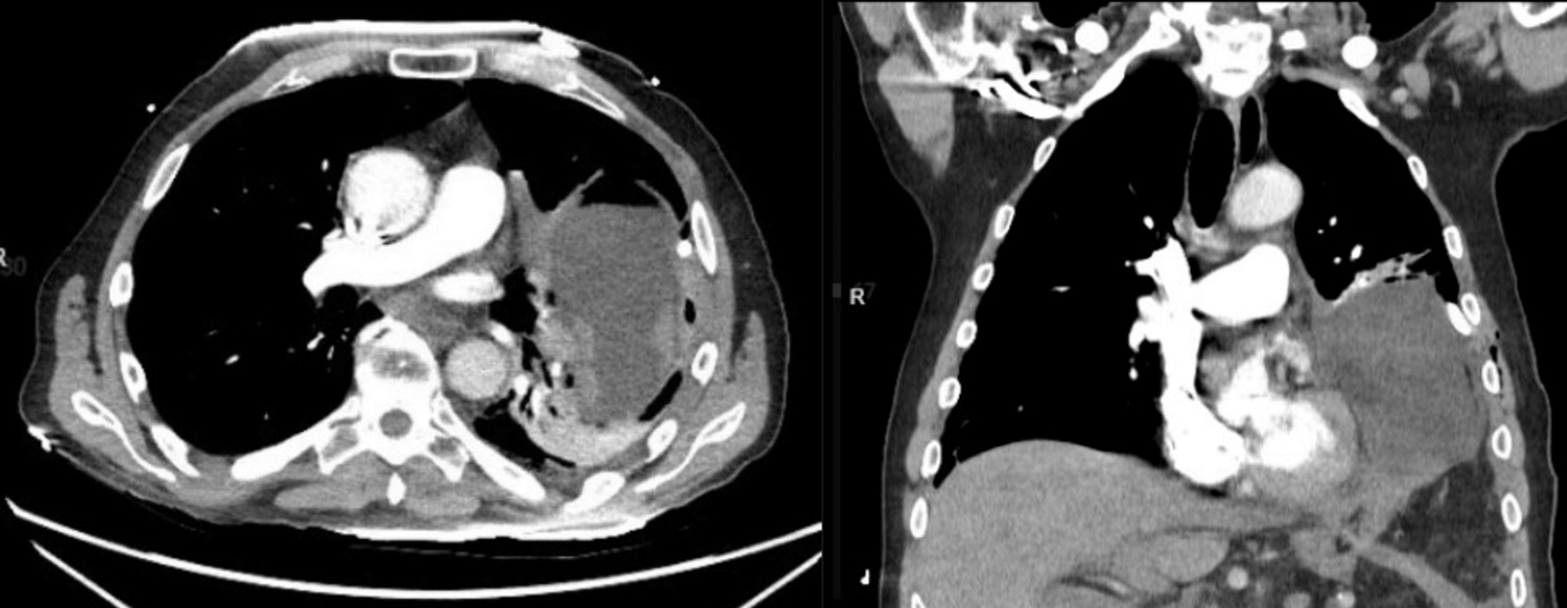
Computed tomography imaging after chest tube insertion showing gastrothorax with adjacent pigtail chest tube CT imaging showing the stomach in the left hemithorax with a chest tube coursing laterally to the stomach.
